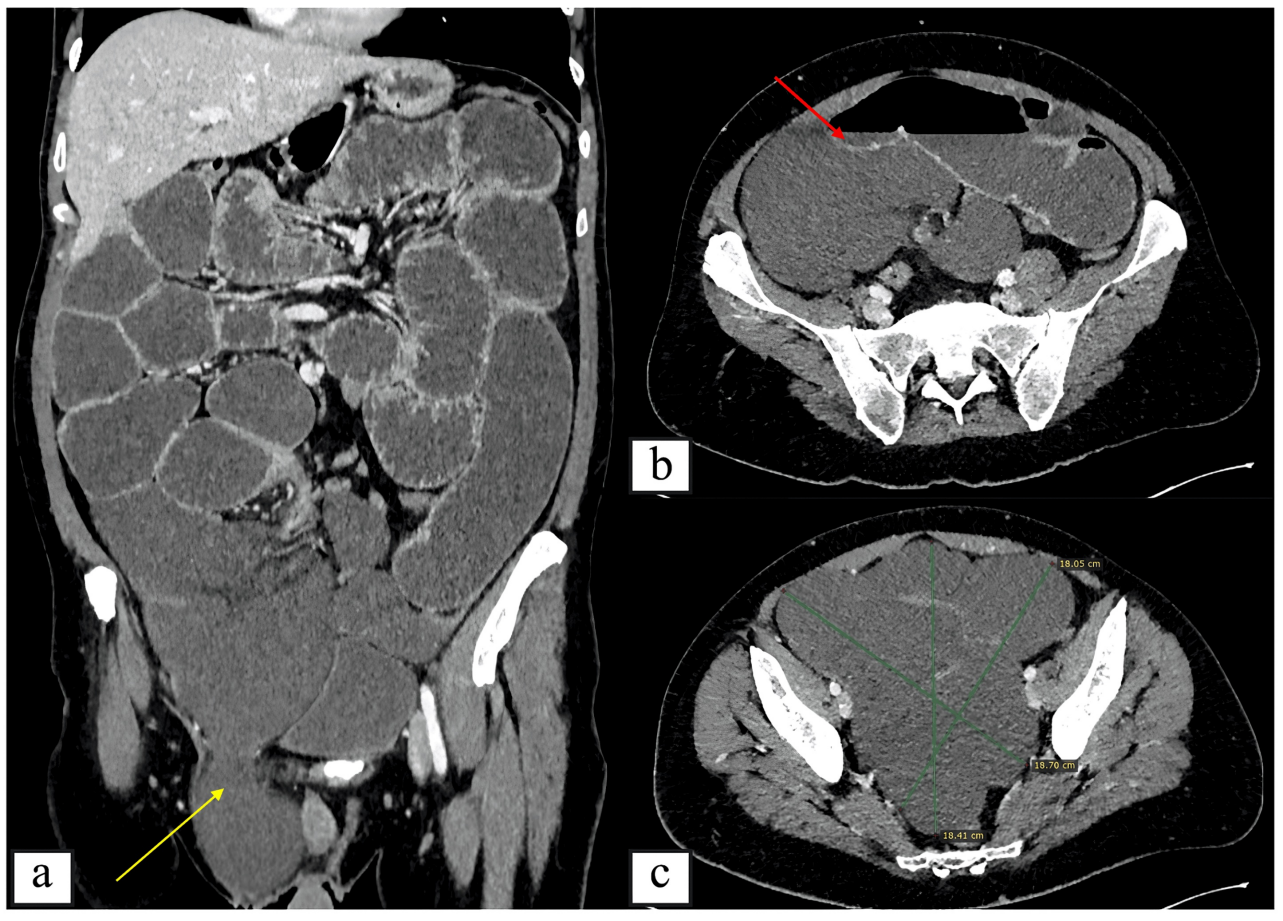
Contrast-enhanced CT images of the abdomen (a) Coronal CT image demonstrating a large multicystic lesion in the lower right abdomen protruding into a right inguinal hernia (yellow arrow). (b) Axial (transverse) CT images demonstrating differences in enhancement between the small bowel wall (red arrow) and the cystic lesion, as well as the overall size of the multiloculated mass measuring approximately 18.3 × 18.7 × 18.4 cm. (c) Axial contrast-enhanced CT image demonstrating the overall size and extent of the large multiloculated cystic mass, measuring approximately 18.3 × 18.7 × 18.4 cm.
